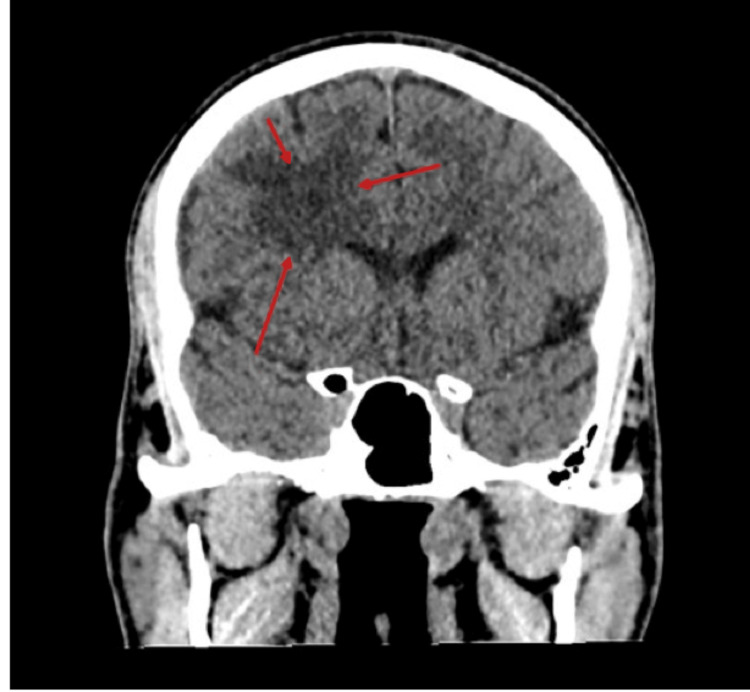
Non-contrast CT of the brain showing a right frontal hypodense lesion Non-contrast axial CT image shows a 3.5 × 3.0 × 2.6 cm hypodense lesion (arrow) in the right anterior frontal lobe white matter. No hemorrhage, significant mass effect, or midline shift is observed. This finding raised initial concern for a demyelinating or cystic process.
